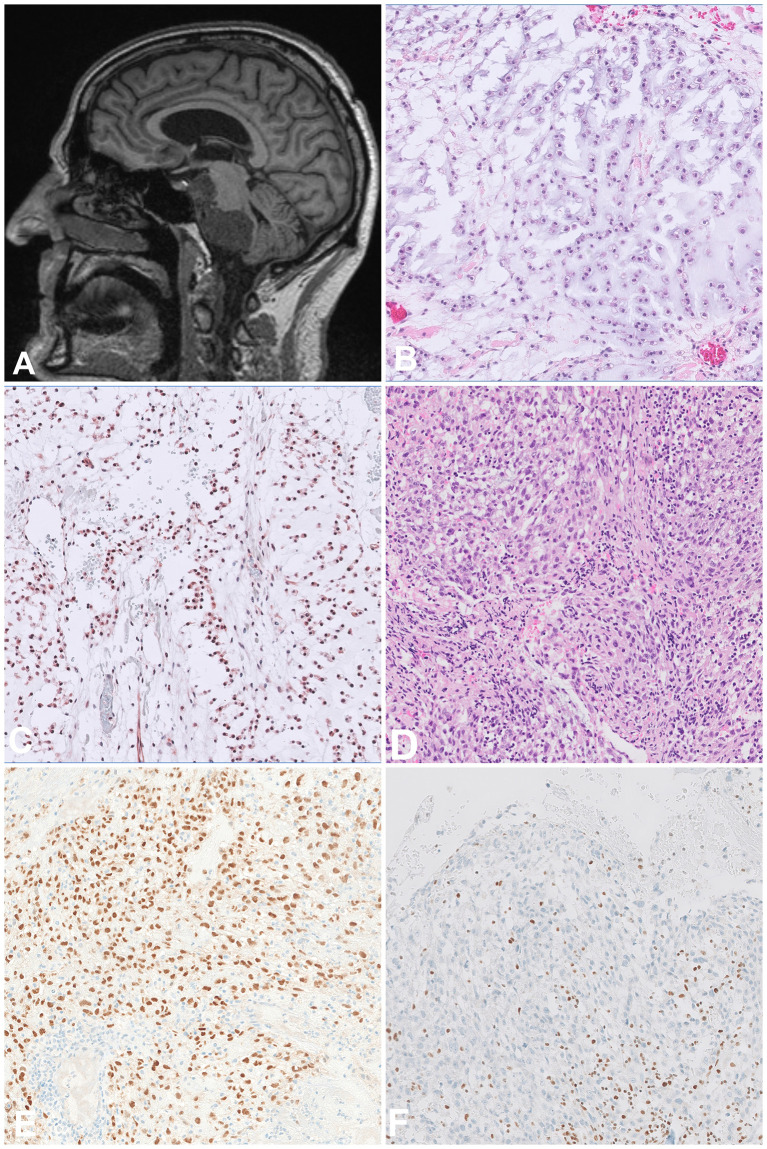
Chordoma. (A) Sagittal MRI scan showing a destructive tumour arising in the skull base and extending to the adjacent structures. (B, C) Conventional chordoma composed of atypical epithelioid cells arranged in chords and set in abundant myxoid matrix with a light basophilic appearance, the cells are positive for Brachyury. (D, E) Poorly differentiated chordoma composed of atypical epithelioid cells with a rhabdoid morphology and set in a more fibrous stroma, the cells are positive for Brachyury. (F) The tumour cells show lost expression of INI1, as opposed to background lymphocytes which can be used as positive control.
